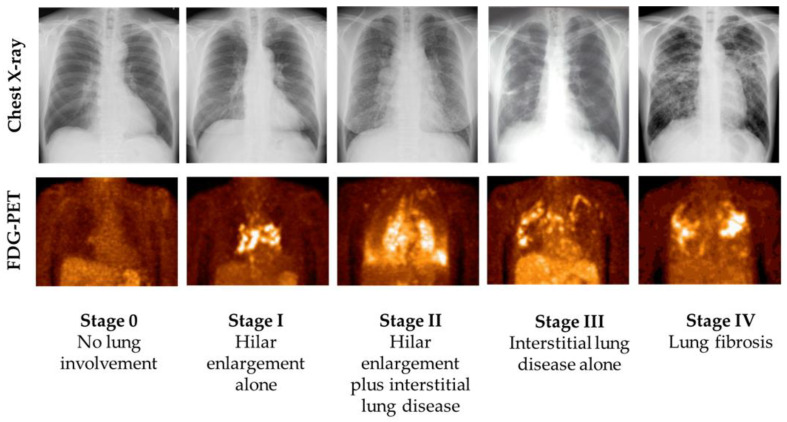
Chest X-ray and FDG-PET images according to the Scadding stage. 18F-Fluorodeoxyglucose-positron emission tomography (FDG-PET) showing FDG uptake in inflammatory lesions of pulmonary sarcoidosis.
